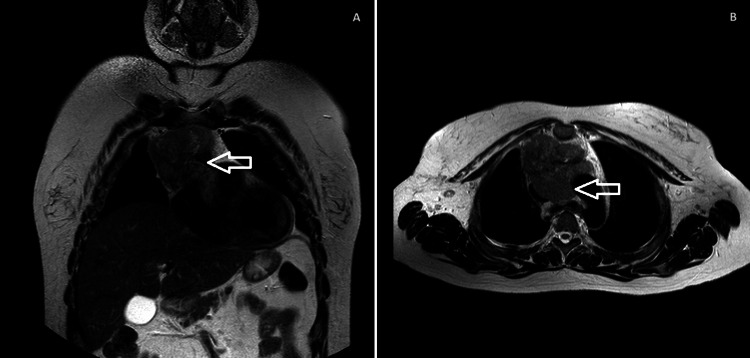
Thoracic magnetic resonance imaging shows coronal (A) and axial (B) views of an anterior mediastinum mass (thymoma) with invasion of the left brachycephalic venous trunk and pericardium. The superior vena cava and ascending aorta are in continuity with the lesion, resulting in significant compression of the superior vena cava.
